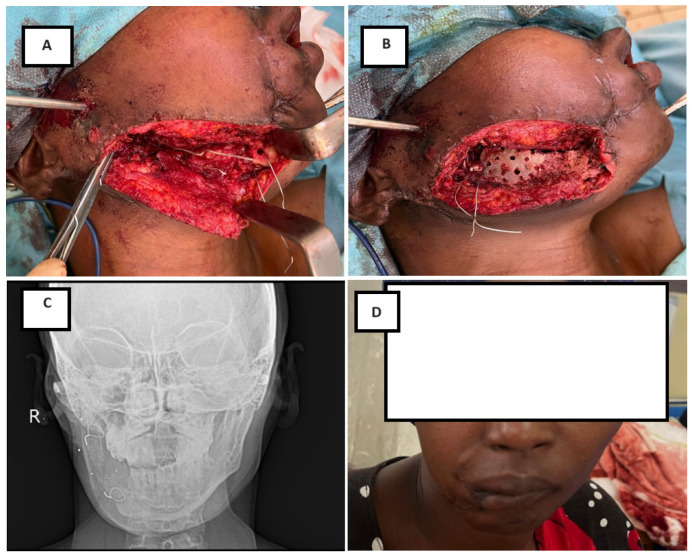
Secondary reconstruction following initial external fixation in the patient presented in Figure 1. (A) Intraoperative view through an extended submandibular approach allowing exposure of the defect and preparation of the recipient site. (B) Corticocancellous iliac crest bone graft shaped to fit the defect and fixed to the mandibular segments using wire fixation. (C) Six-month postoperative radiographic follow-up demonstrating restoration of mandibular continuity. (D) Six-month postoperative clinical appearance.
